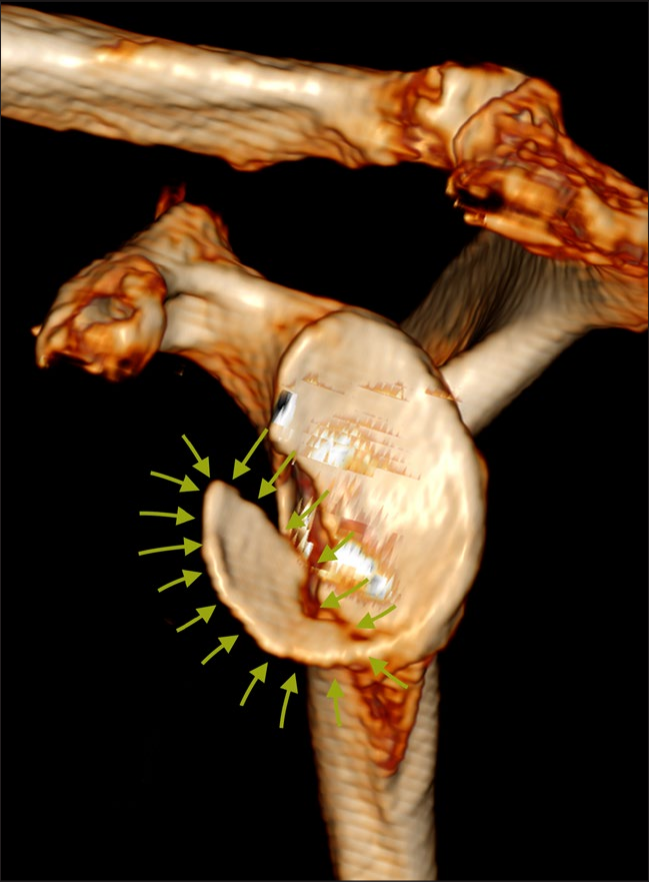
3D MDCT of bony Bankart lesion. 3D volume rendering CT of a large avulsed anteroinferior glenoid bony fragment (outlined by small arrows) using surface rendering and subtraction of humeral head that will be treated by surgical fixation.
